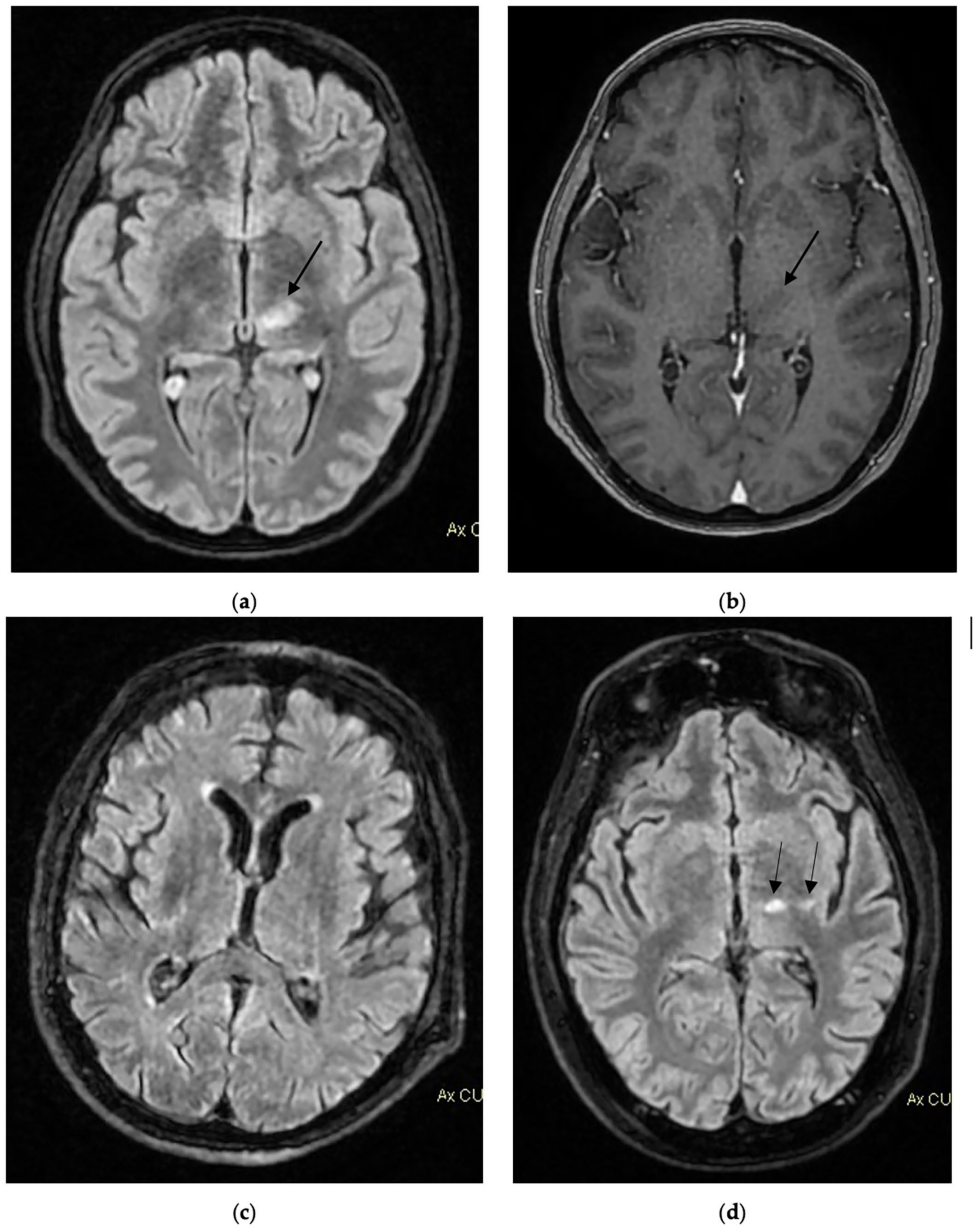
Representative brain MRI images from selected cases: Case 1, two foci of hyperintensity on long TR sequences (a) and axial T1 gradient echo contrast-enhanced (b)—one in the left thalamus (14 × 8 mm), showing no contrast enhancement; Case 2, (c) Axial T2/FLAIR image demonstrating small areas of hyperintensity on long TR sequences, located in the deep periventricular white matter bilaterally and in the corona radiata (semioval centers); Case 3, (d) Axial T2/FLAIR image showing two subcentimetric pseudonodular lesions in the left thalamus and internal capsule region, appearing hyperintense on long TR sequences.
